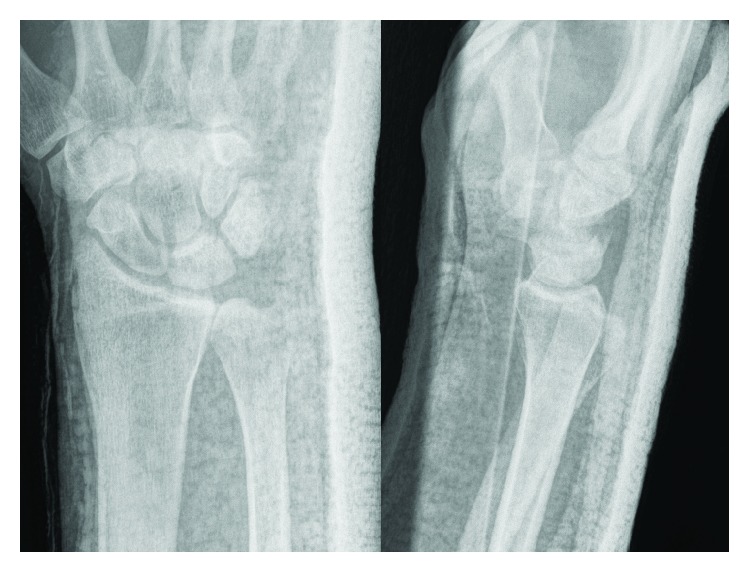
Postreduction radiographs of the right wrist. There is no more overlap between the radius and the ulna on the AP view, and the ulnar head is now slightly dorsal to the radius on the lateral view.
